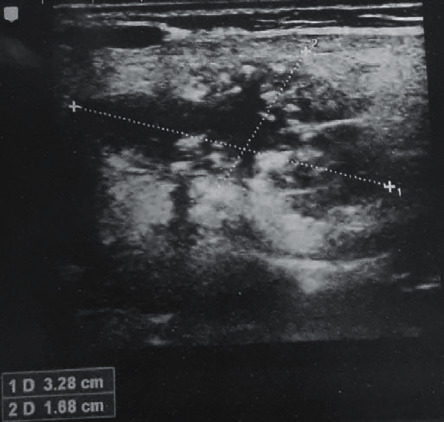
Picture showing ultrasound imaging, suggestive of arteriovenous malformation.
